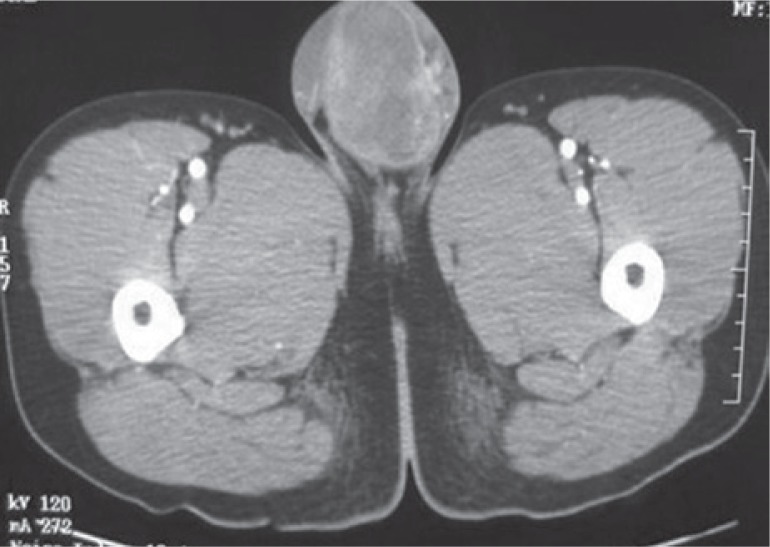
CT scan of the scrotum demonstrating a large hyperdense mass measuring ∼5.5×5 cm in the right testicular region. CT, computed tomography.
